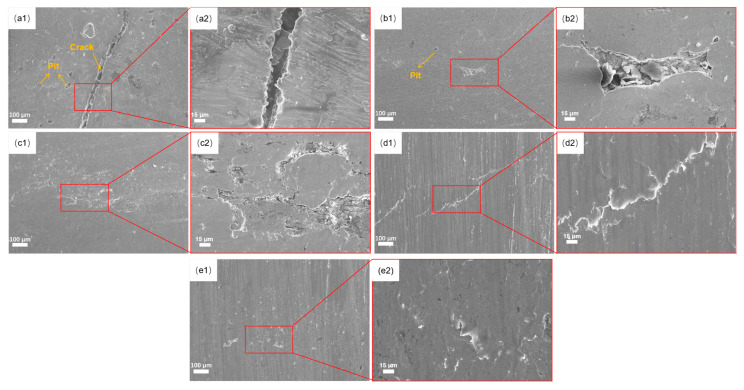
Surface morphology of coatings with different indentations at 0.3 MPa: (a1,a2) no ultrasonic rolling, (b1,b2) rolling depth of 0.05 mm, (c1,c2) rolling depth of 0.085 mm, (d1,d2) rolling depth of 0.125 mm, (e1,e2) rolling depth of 0.15 mm.
